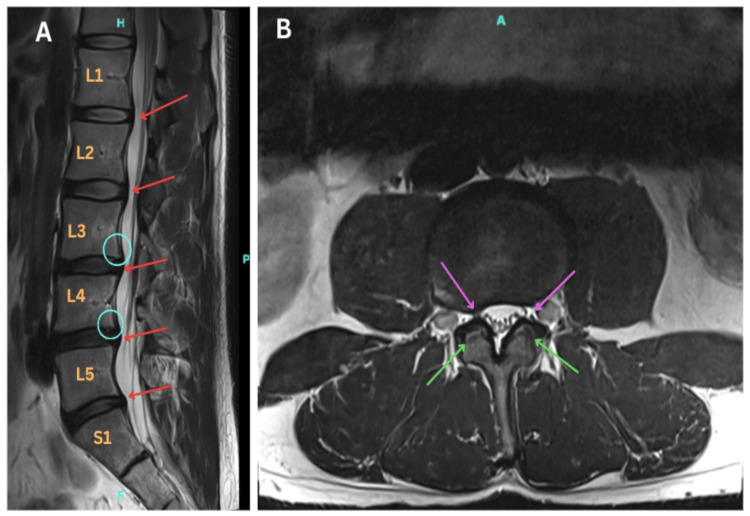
Magnetic resonance imaging of the lumbar spine without contrast (A) Sagittal T2‑weighted image demonstrates multilevel degenerative disc disease; blue circles indicate retrolisthesis at L3‑L4 and L4‑L5, and red arrows mark moderate spinal canal stenosis from L2 through L5. (B) Axial T2‑weighted image at the L4‑L5 level; purple arrows highlight bilateral foraminal narrowing, and green arrows indicate facet arthropathy.
